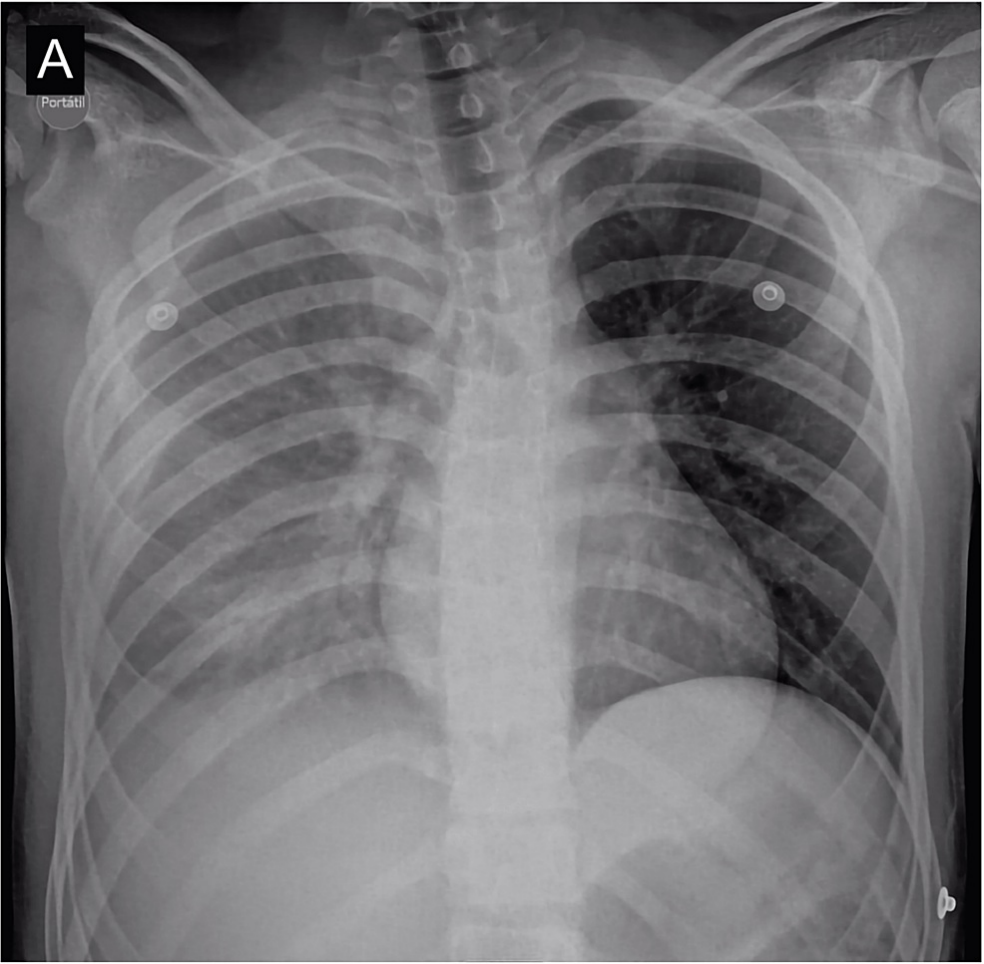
Anteroposterior chest x-ray, shows diffuse increase in the opacity of the right hemithorax due to pleural effusion.
